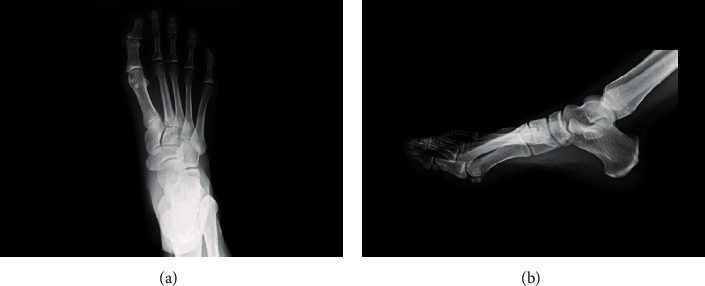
Preoperative foot radiograph at an initial visit to our institution. An oblique fracture line at the base of the second metatarsal was identified on (a) anteroposterior and (b) lateral views.
